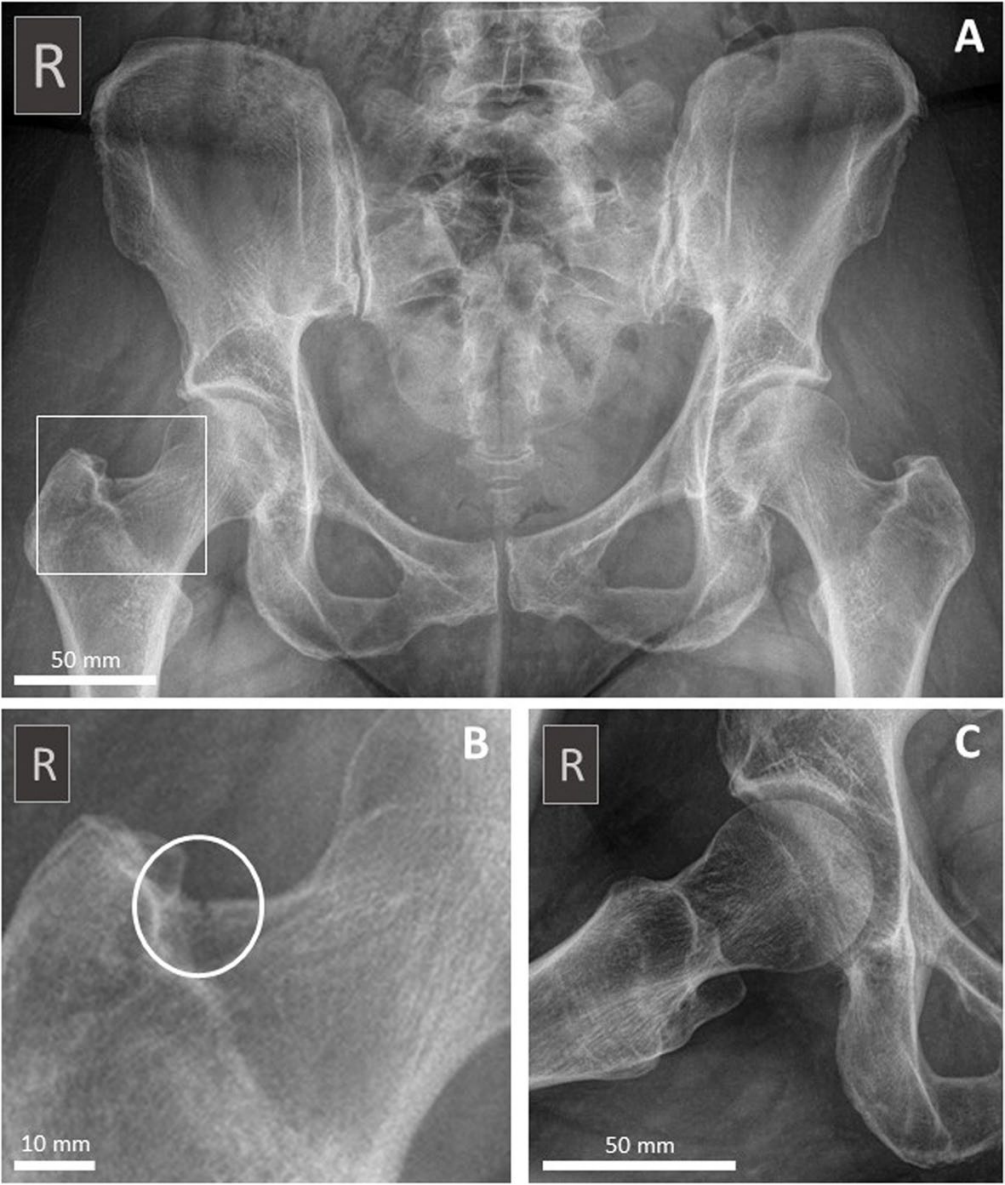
Discrete hip fracture on conventional X-rays. A 59-year-old female consulting a chiropractic clinic with idiopathic, ongoing debilitating right-sided groin pain through four weeks, gradually increasing severity despite intensive physiotherapy rehabilitation. An in-house standing X-ray examination of the pelvis (AP projection (A), closeup of the right hip from A (B) and frog leg view (C)) was performed showing generalized osteopenic appearance of the bone and a discrete fracture (white circle in B) through the cortex of upper part of the femoral neck on the right side. The patient was referred to the hospital where stabilizing surgery was performed. Medical treatment for DEXA-confirmed osteoporosis was initiated
